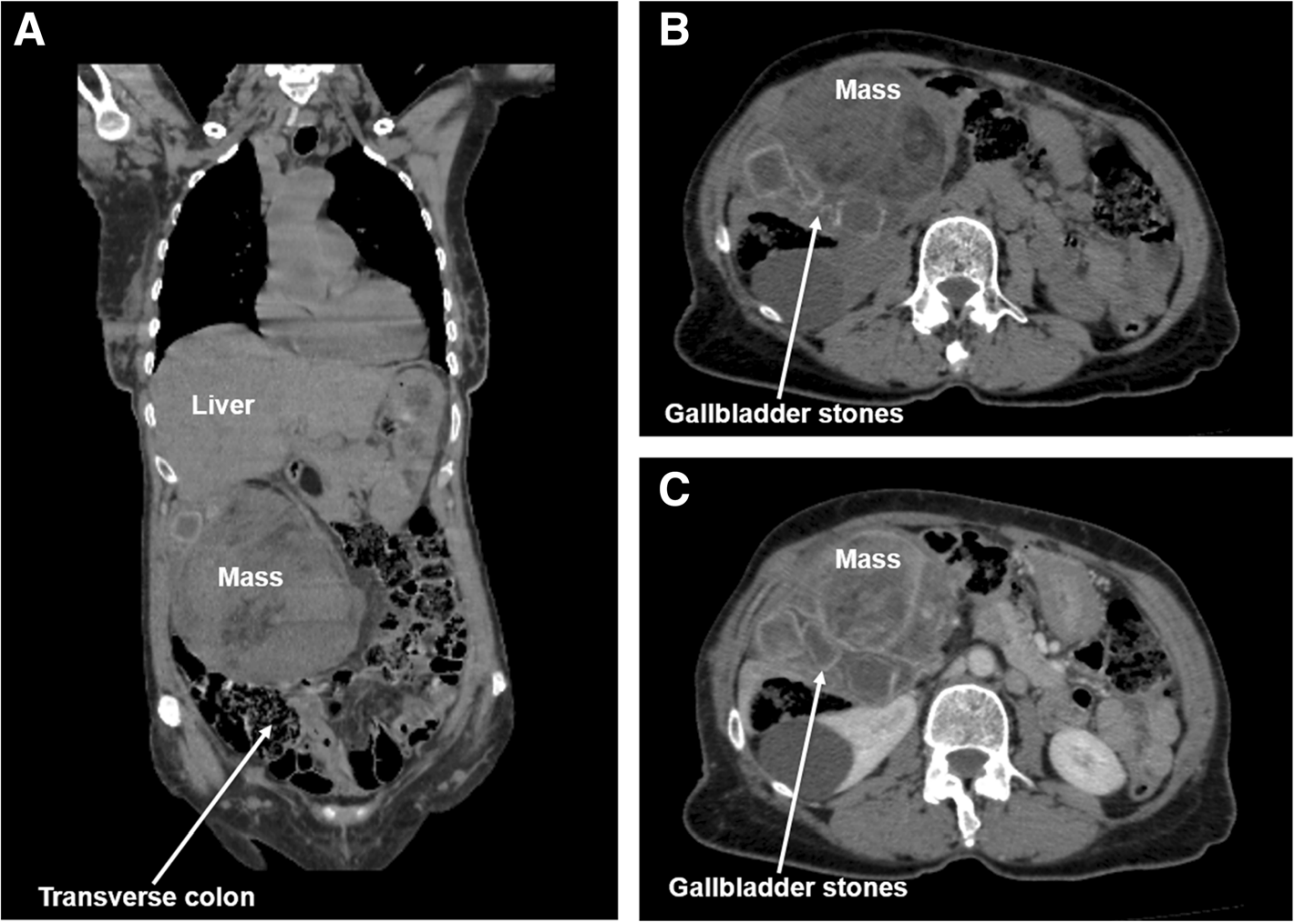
Preoperative CT scans: coronal section of non-enhanced CT image showing a large mass adhered to the liver, pushing the transverse colon down (a). Transverse section imaging showing three large gallbladder stones without (b) and with (c) CT contrast enhancement
